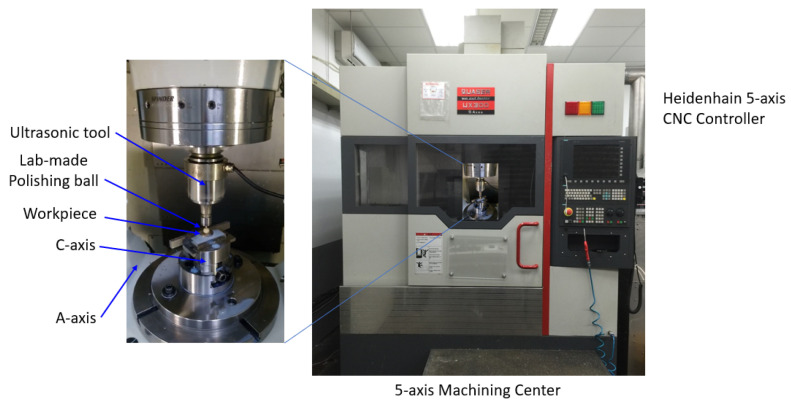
Photo of the ultrasonic vibration-assisted ball-polishing tool mounted on the 5-axis machining center.
